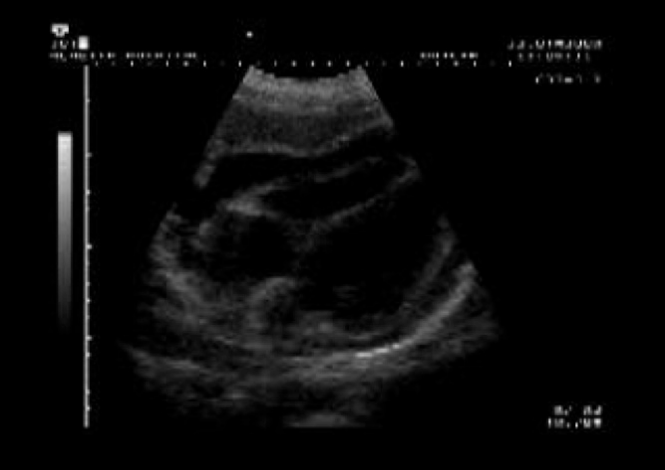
Ultrasound on day 3 for the patient, showing reduction in pericardial effusion.
